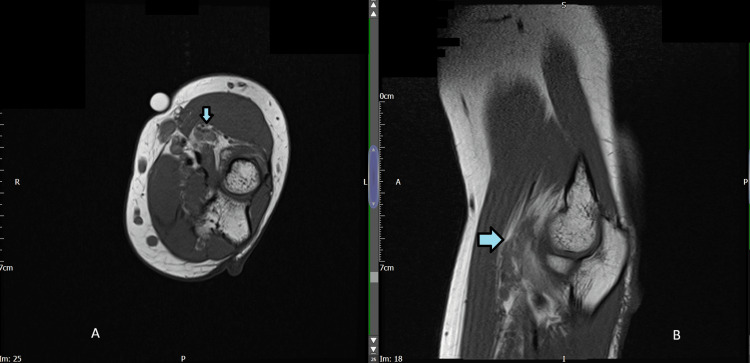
T1 Weighted MRI images of the patient T1-weighted MRI images at the level of the radial head with the lymphovascular malformation highlighted by arrows. Image A (axial) and image B (sagittal).
